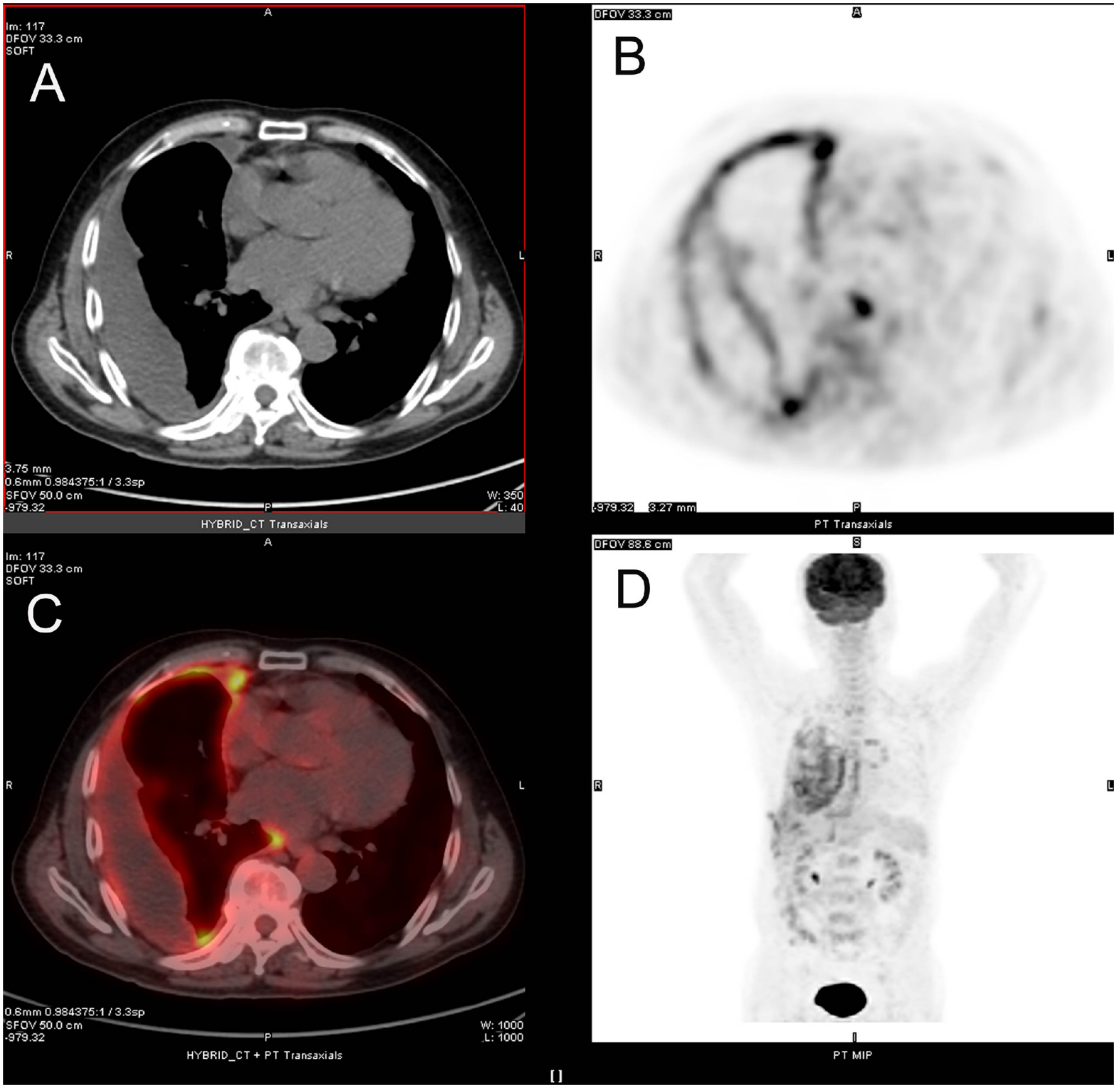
^18^F-FDG PET/CT integrated imaging of 72-year old man with tuberculous pleural effusion. Axial CT (A) shows effusion in right pleural cavity and diffuse light smooth thickening of the pleura. Axial ^18^F-FDG PET (B) and axial fused ^18^F-FDG PET/CT (C) display diffuse ^18^F-FDG uptake in right pleural region (SUVmax of 7.9).
